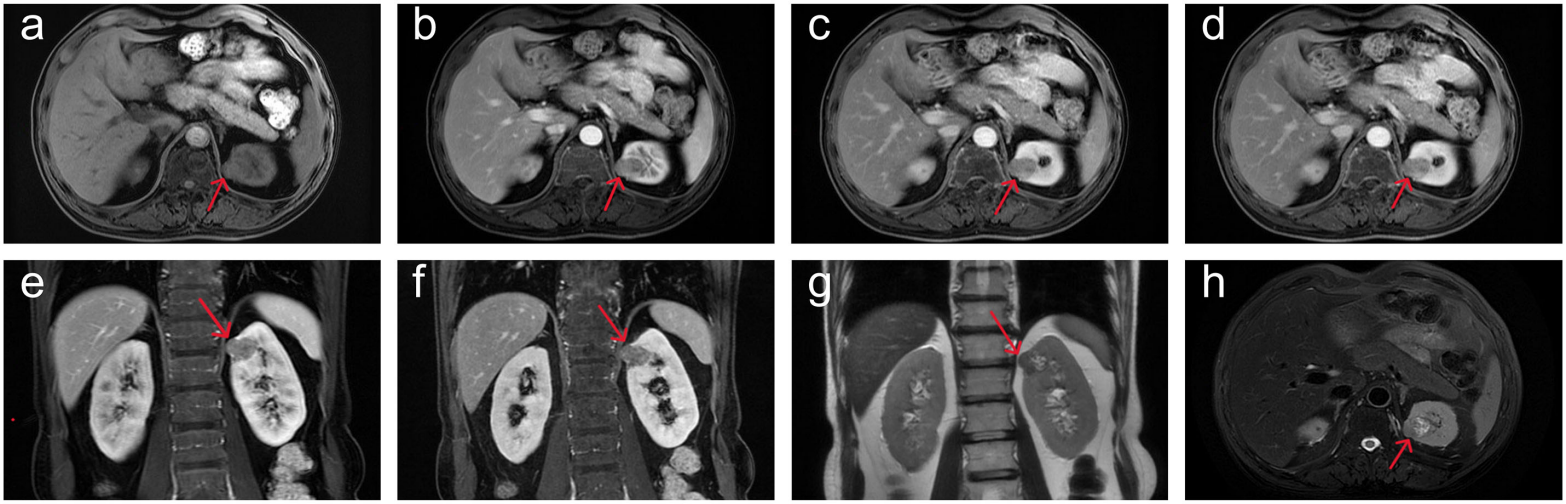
Preoperative renal MRI showed round-like abnormal signal in the upper medial pole of the left kidney. (a-f) On contrast-enhanced scan (plain scan, arterial phase, venous phase and delayed phase), the lesions showed mild inhomogeneous enhancement and iso-signal on T1WI. (g, h) T2WI showed iso-high mixed signal.
